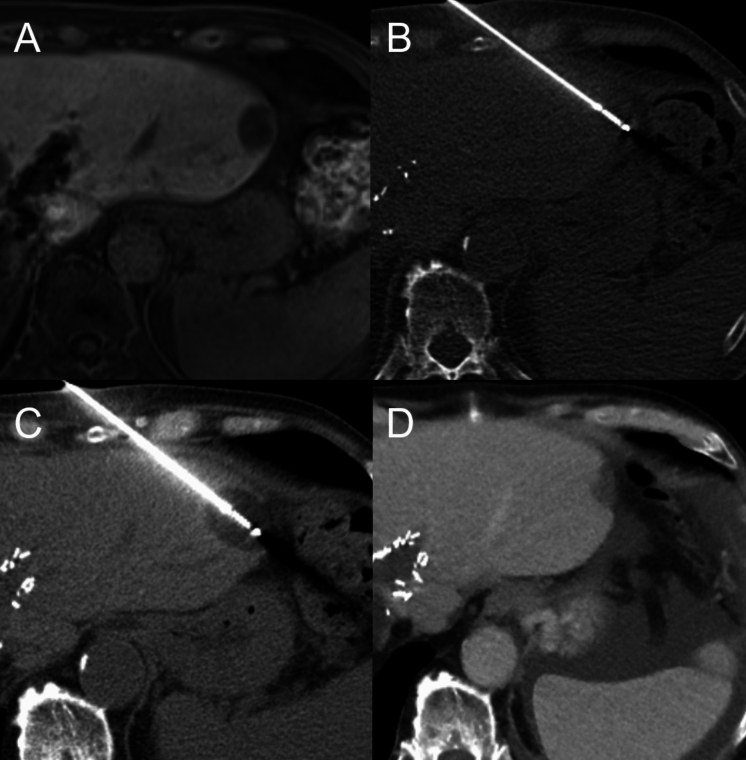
72-year-old male patient with recurrence of a colorectal liver metastasis in the left liver lobe. A Preinterventional axial MRI (hepatobiliary phase) shows a single recurrent metastasis in the left liver lobe located directly adjacent to the left colon. B Intraprocedural fluoroscopic placement of a cryo-needle directly into the lesion. C Intraprocedural non-contrast CT scan obtained after 5 min during the second freezing cycle with good intraprocedural visualization of the ice ball. D Follow-up CT (portal venous phase) after 6 months showing local tumor control and intact adjacent intestinal structures. There were no complications
